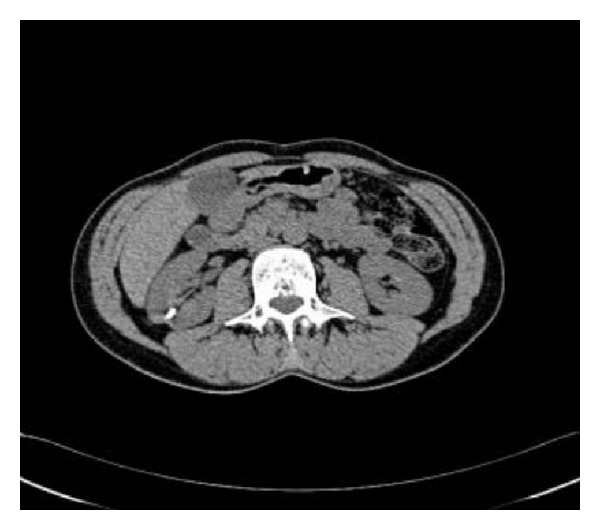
CT scan. Right calyx stone.
